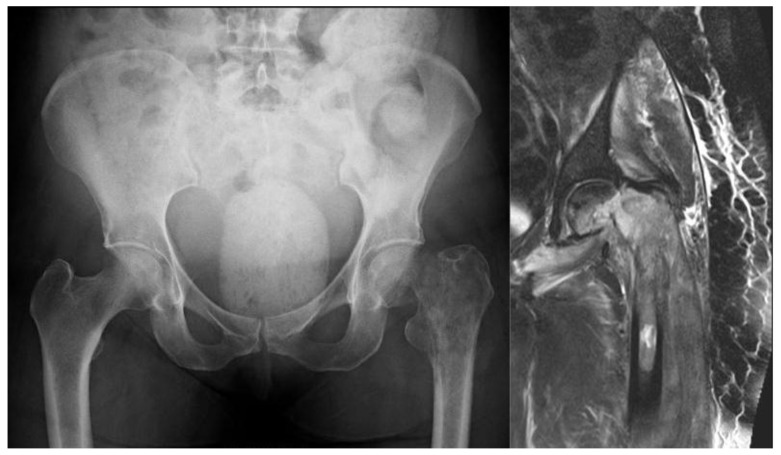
X-ray and MRI showing pathological fracture with involvement of proximal femoral diaphysis.
